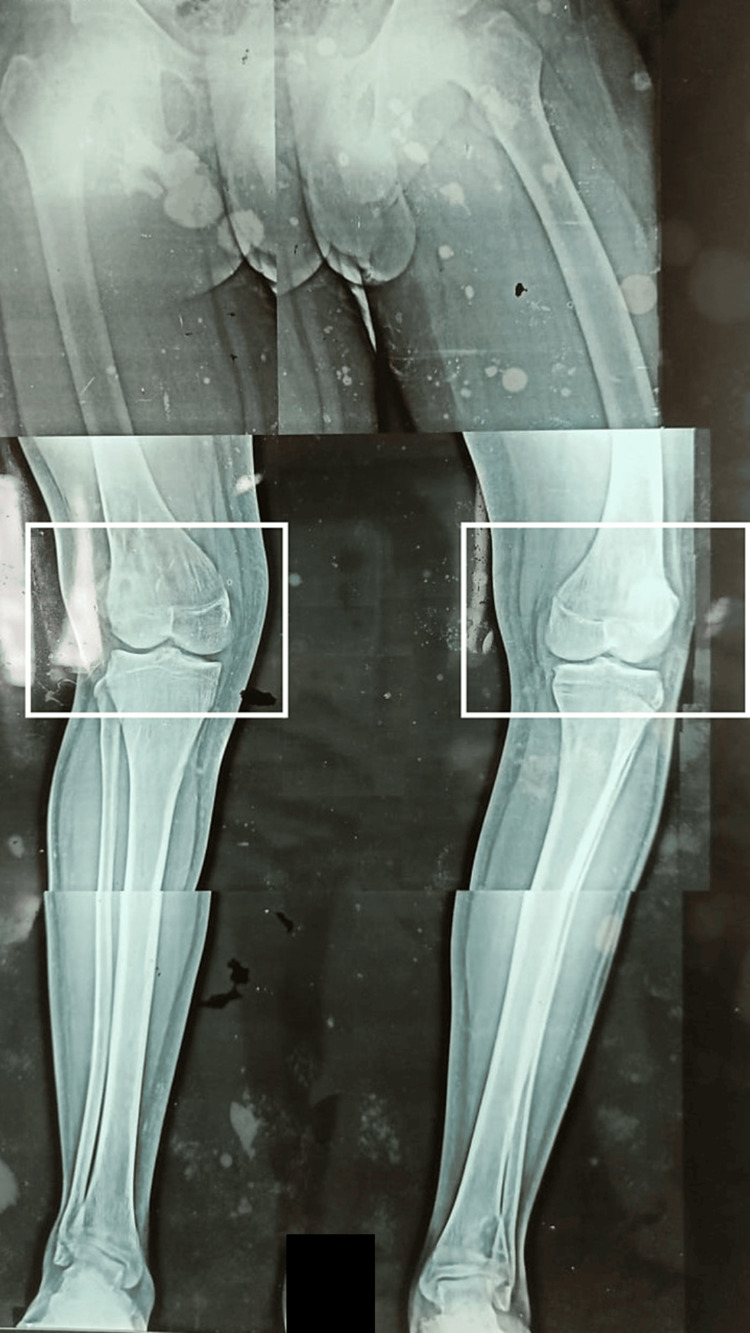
X-ray of bilateral knee joint The rectangle shows genu valgus in the right knee and genu varus in the left knee. The image is a combination of three different X-rays
